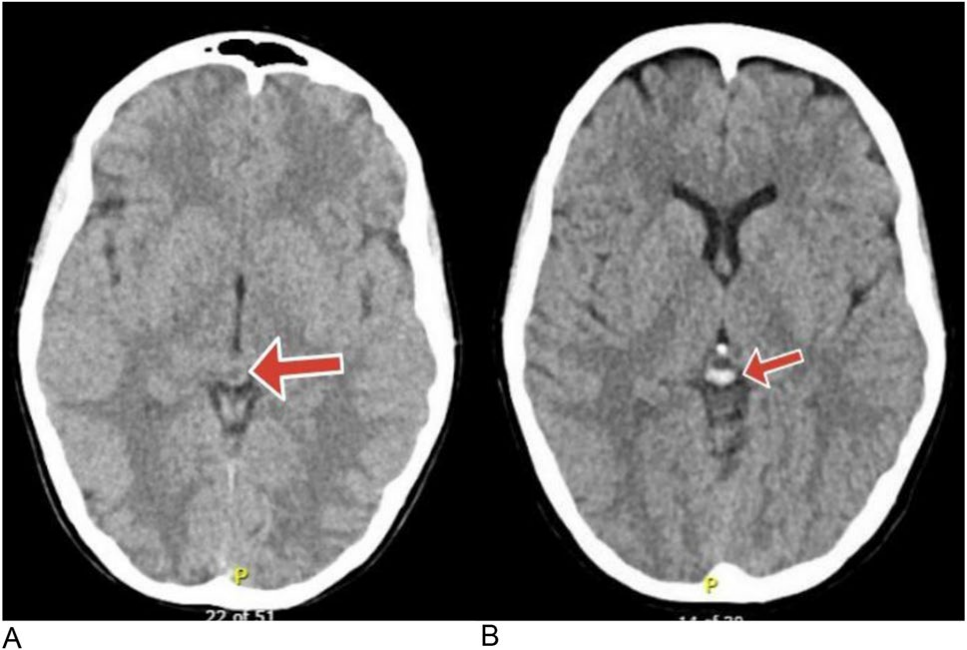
CT brain without contrast showing (A) Pineal cyst accidentally discovered seven years ago. (B) Hyperdense hemorrhagic blood products inside the pineal cyst
